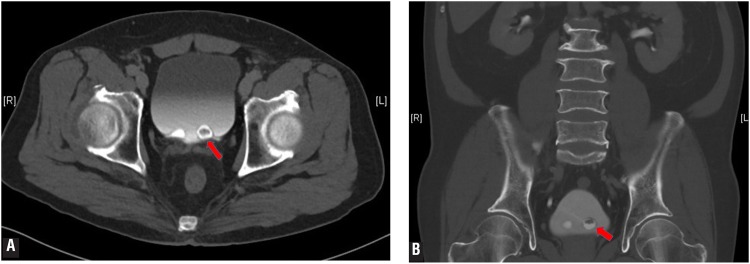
A and B) CT Urography revealed bilateral simple ureteroceles with the left containing a slightly enhancing soft tissue nodule measuring 0.4 × 0.7 × 0.9cm (red arrow).
